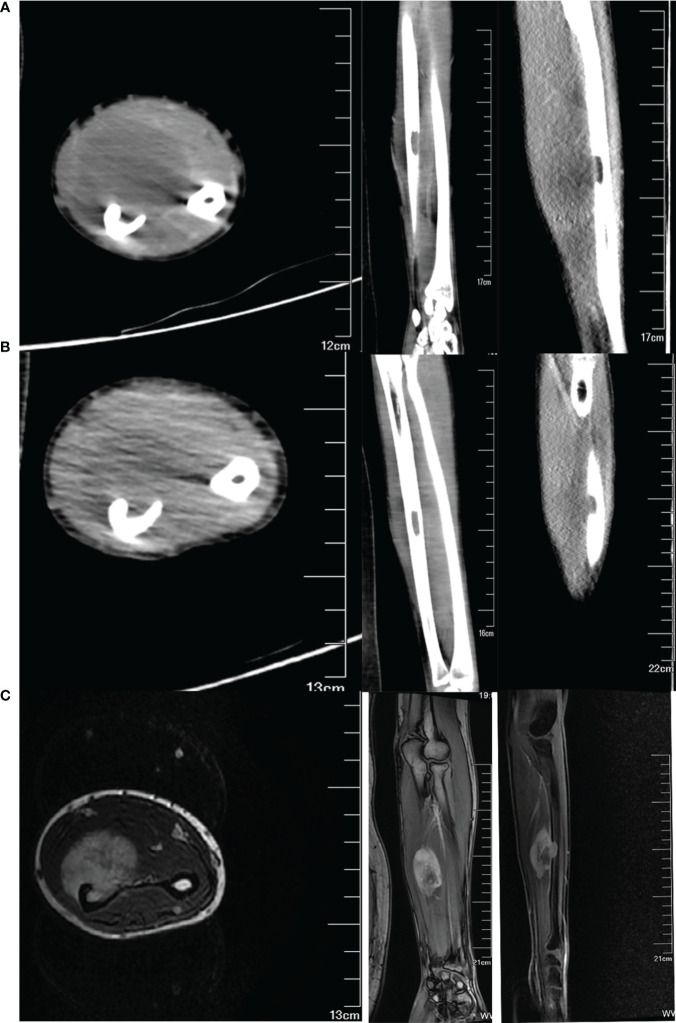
Imaging data of the tumor pre- and post-chemotherapy treatment. (A) CT images of the tumor before chemotherapy in transverse, coronal, and sagittal position. (B) CT images of the tumor after two courses of chemotherapy. (C) T1-weighted MR images of the tumor after two courses of chemotherapy.
